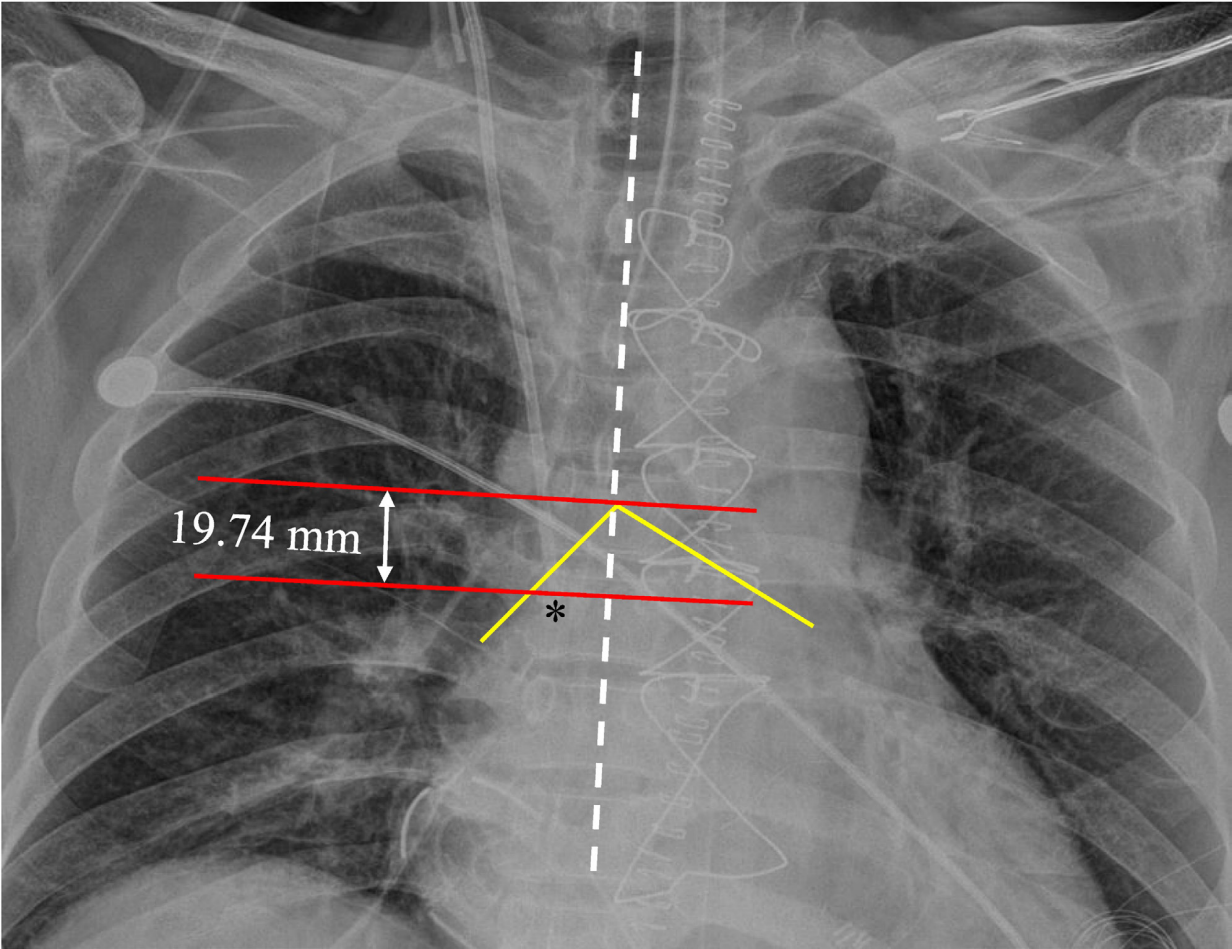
The vertical distance from the catheter tip to the carina measured on postoperative chest X-ray. On the day after the operation, we measured the distance from the catheter tip to the carina using the following steps. Step (1); we drew a vertical line (the white dotted line) parallel to the patient's spine. Step (2); we drew two horizontal lines at the level of the catheter tip and at the level of the carina (red lines) perpendicular to the vertical line. Step (3); we measured the vertical distance between the two horizontal lines. The intersection point of the two yellow lines represents the carina. The asterisk indicates the tip of the central venous catheter.
